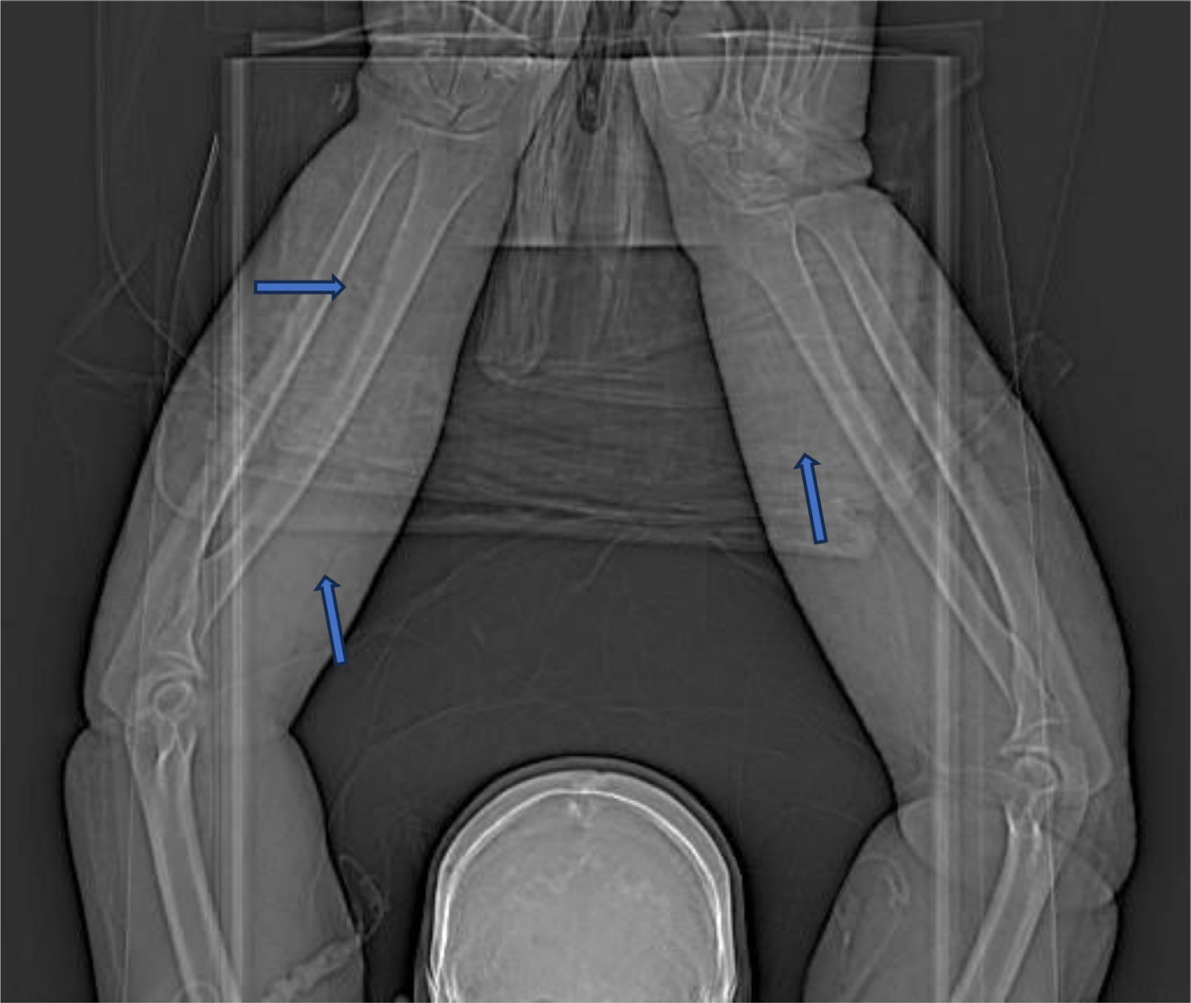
CT Scanogram demonstrating several linear high densities (blue arrows).
